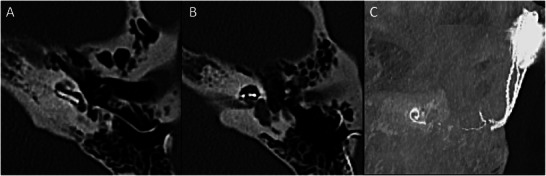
Cone‐beam computed tomography (CBCT) images of a left cochlear implant in situ. Axial CBCT images demonstrate the cochlear lead entering the mastoid bowl and round window to the basal turn (A) and middle turn (B) of the cochlea. (C) A 3D maximum‐intensity projection of the CBCT images demonstrates the position of the cochlear implant on a coronal oblique view.
